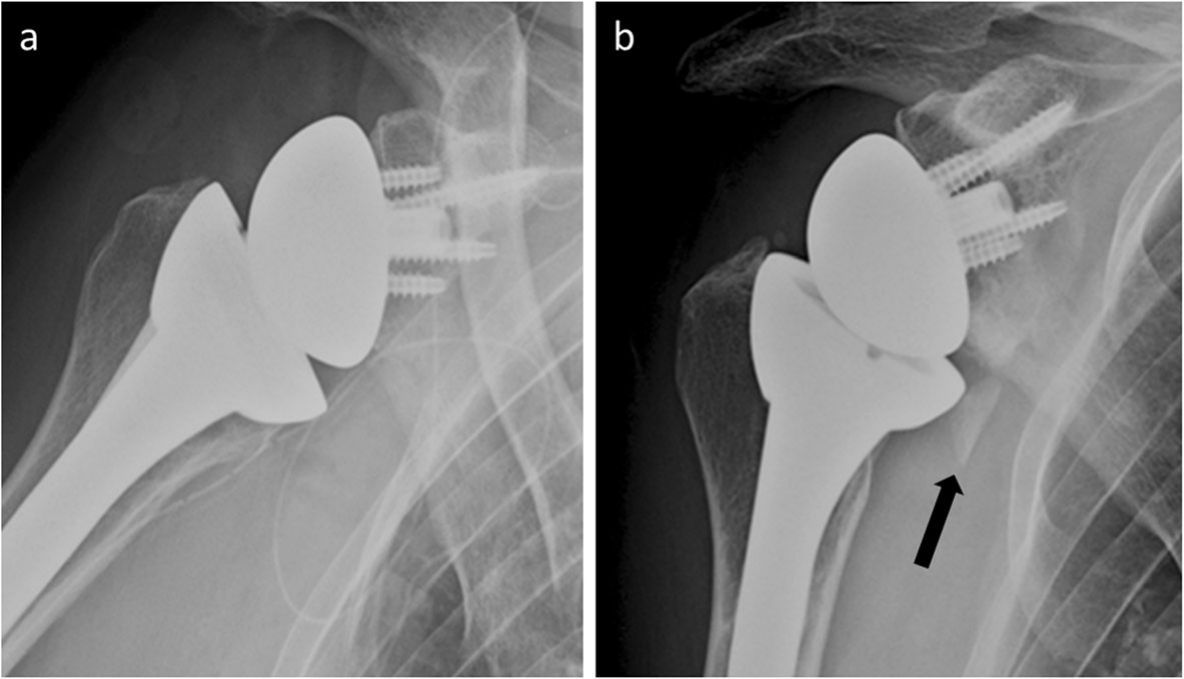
Anteroposterior shoulder radiographs of an 84-year-old woman with reverse shoulder arthroplasty. a Normal postoperative radiograph. b One year later, radiograph shows heterotopic ossification (arrow), with the patient asymptomatic
